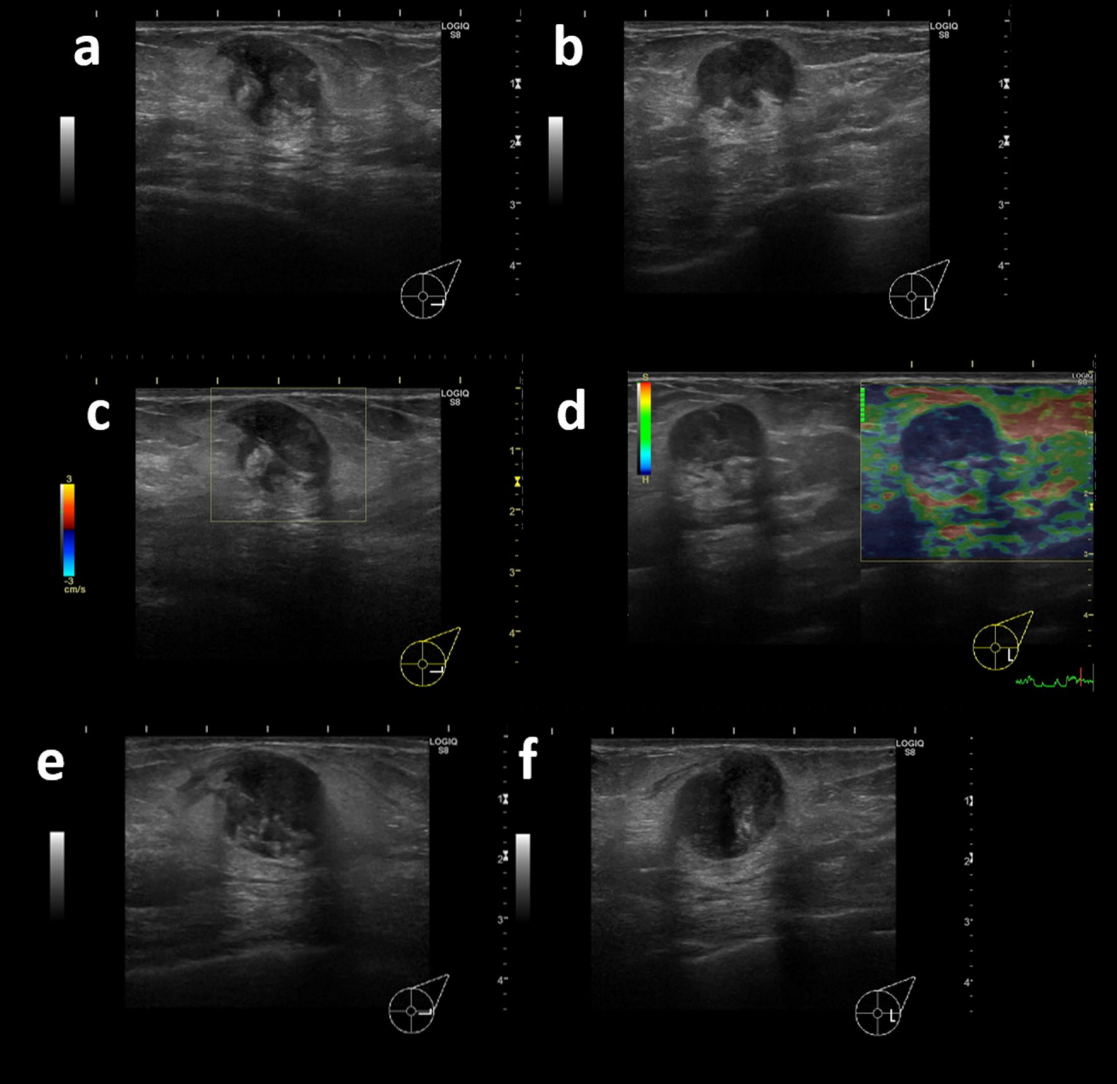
Breast ultrasonography. a US reveals a solid, round mass with circumscribed margins in the lower outer quadrant of the left breast at 4 o'clock, measuring 16 × 16 × 15 mm. The internal echo of the tumor was a mixture of relatively uniform hypoechoic areas with posterior enhancement, and heterogeneous hyperechoic areas. Color Doppler showed no blood flow in the tumor. b At 2 months follow-up ultrasonography, revealed an increase in the size of the mass to 27 × 26 × 19-mm
